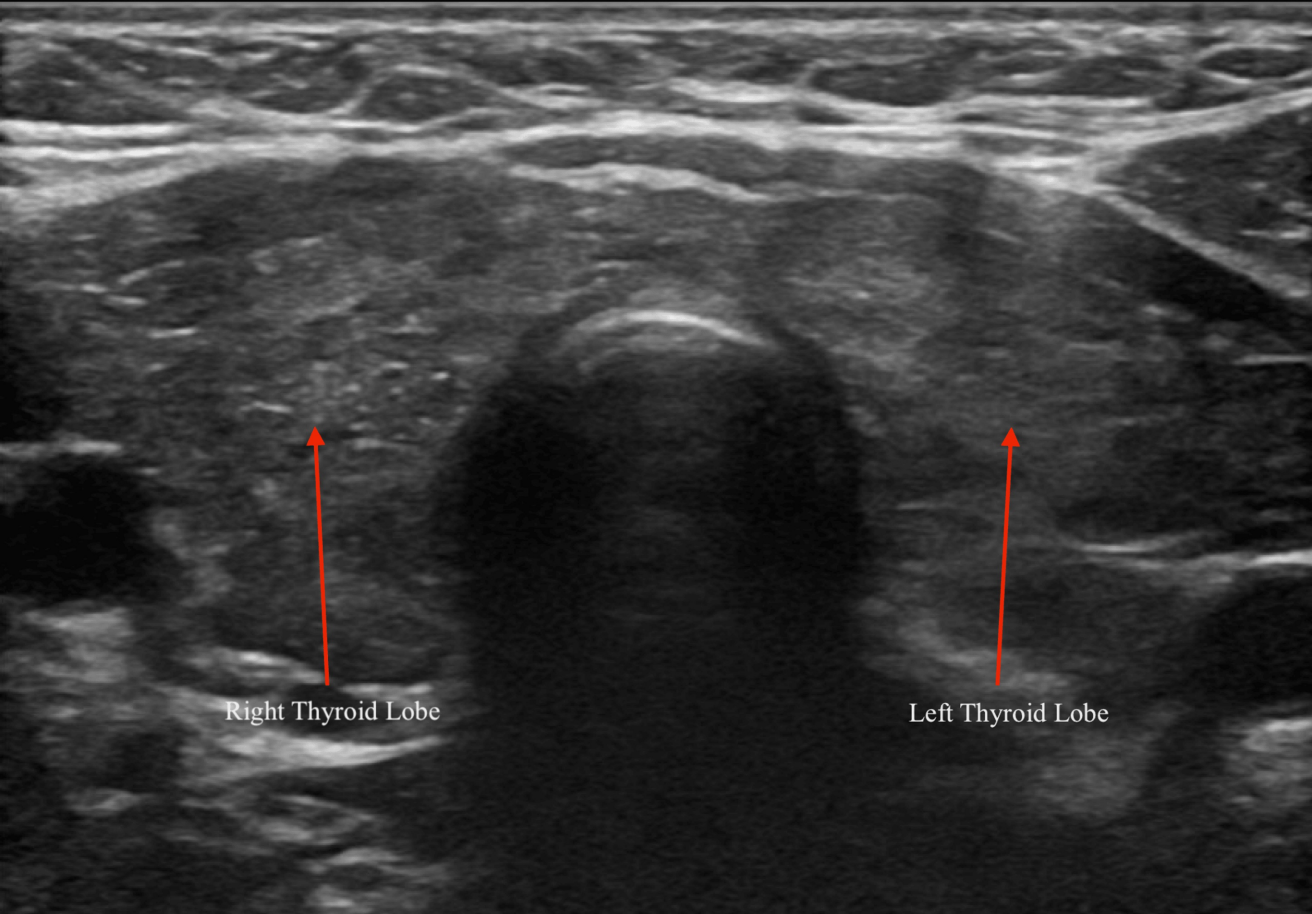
Transverse Thyroid Ultrasound Demonstrating No Abnormalities
